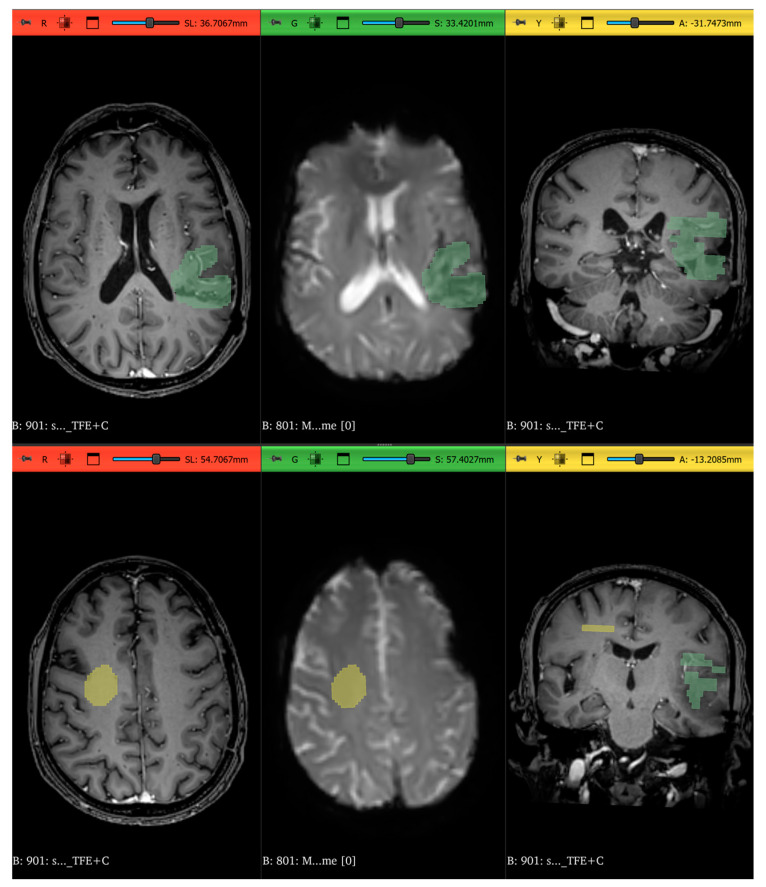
Two samples (top and bottom) of the segmentation process using 3D Slicer^®^. The segmentation of the region of interest of radionecrosis or tumor progression can be seen in green and the segmentation of the NAWM in yellow. (Left): axial contrast-enhanced T1-weighted image. (Middle): axial DSC MRI perfusion sequence. (Right): coronal contrast-enhanced T1-weighted image.
